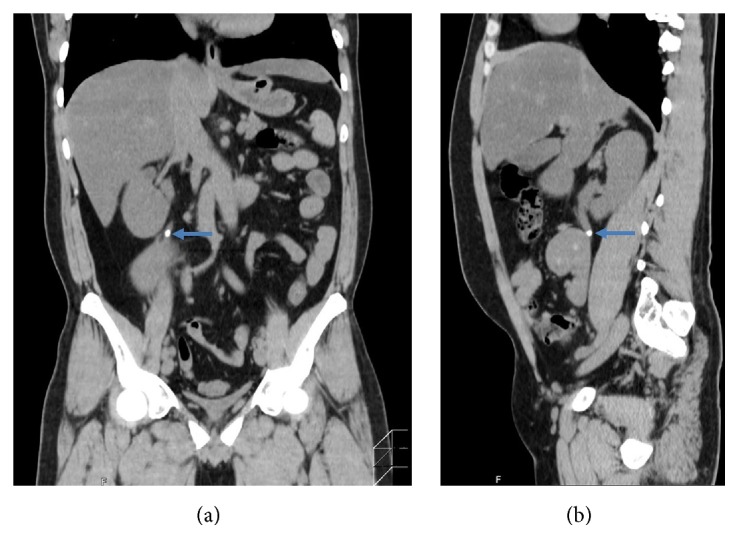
Coronal (a) and sagittal (b) sections of unenhanced computed tomography of the abdomen show left-to-right crossed unfused renal ectopia and a 5 mm stone (arrows) in the proximal ureter of the kidney located cranially. A left inferior vena cava is also seen.
